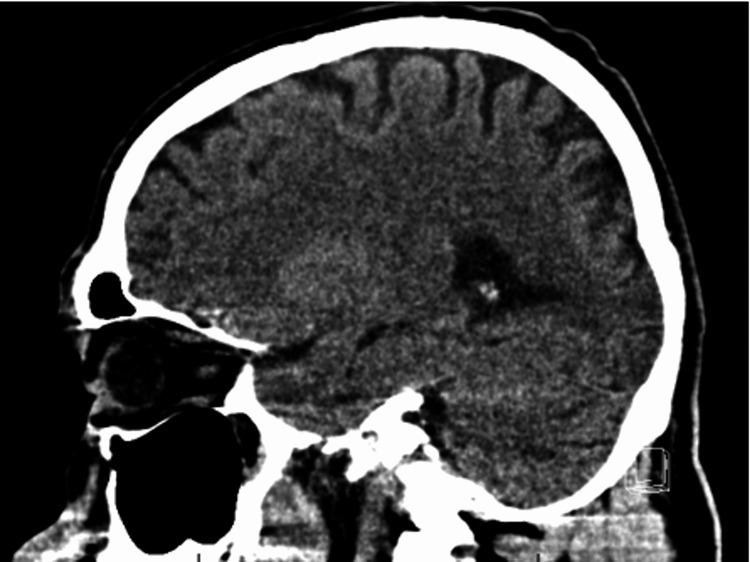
Normal brain CT without contrast (sagittal view)
